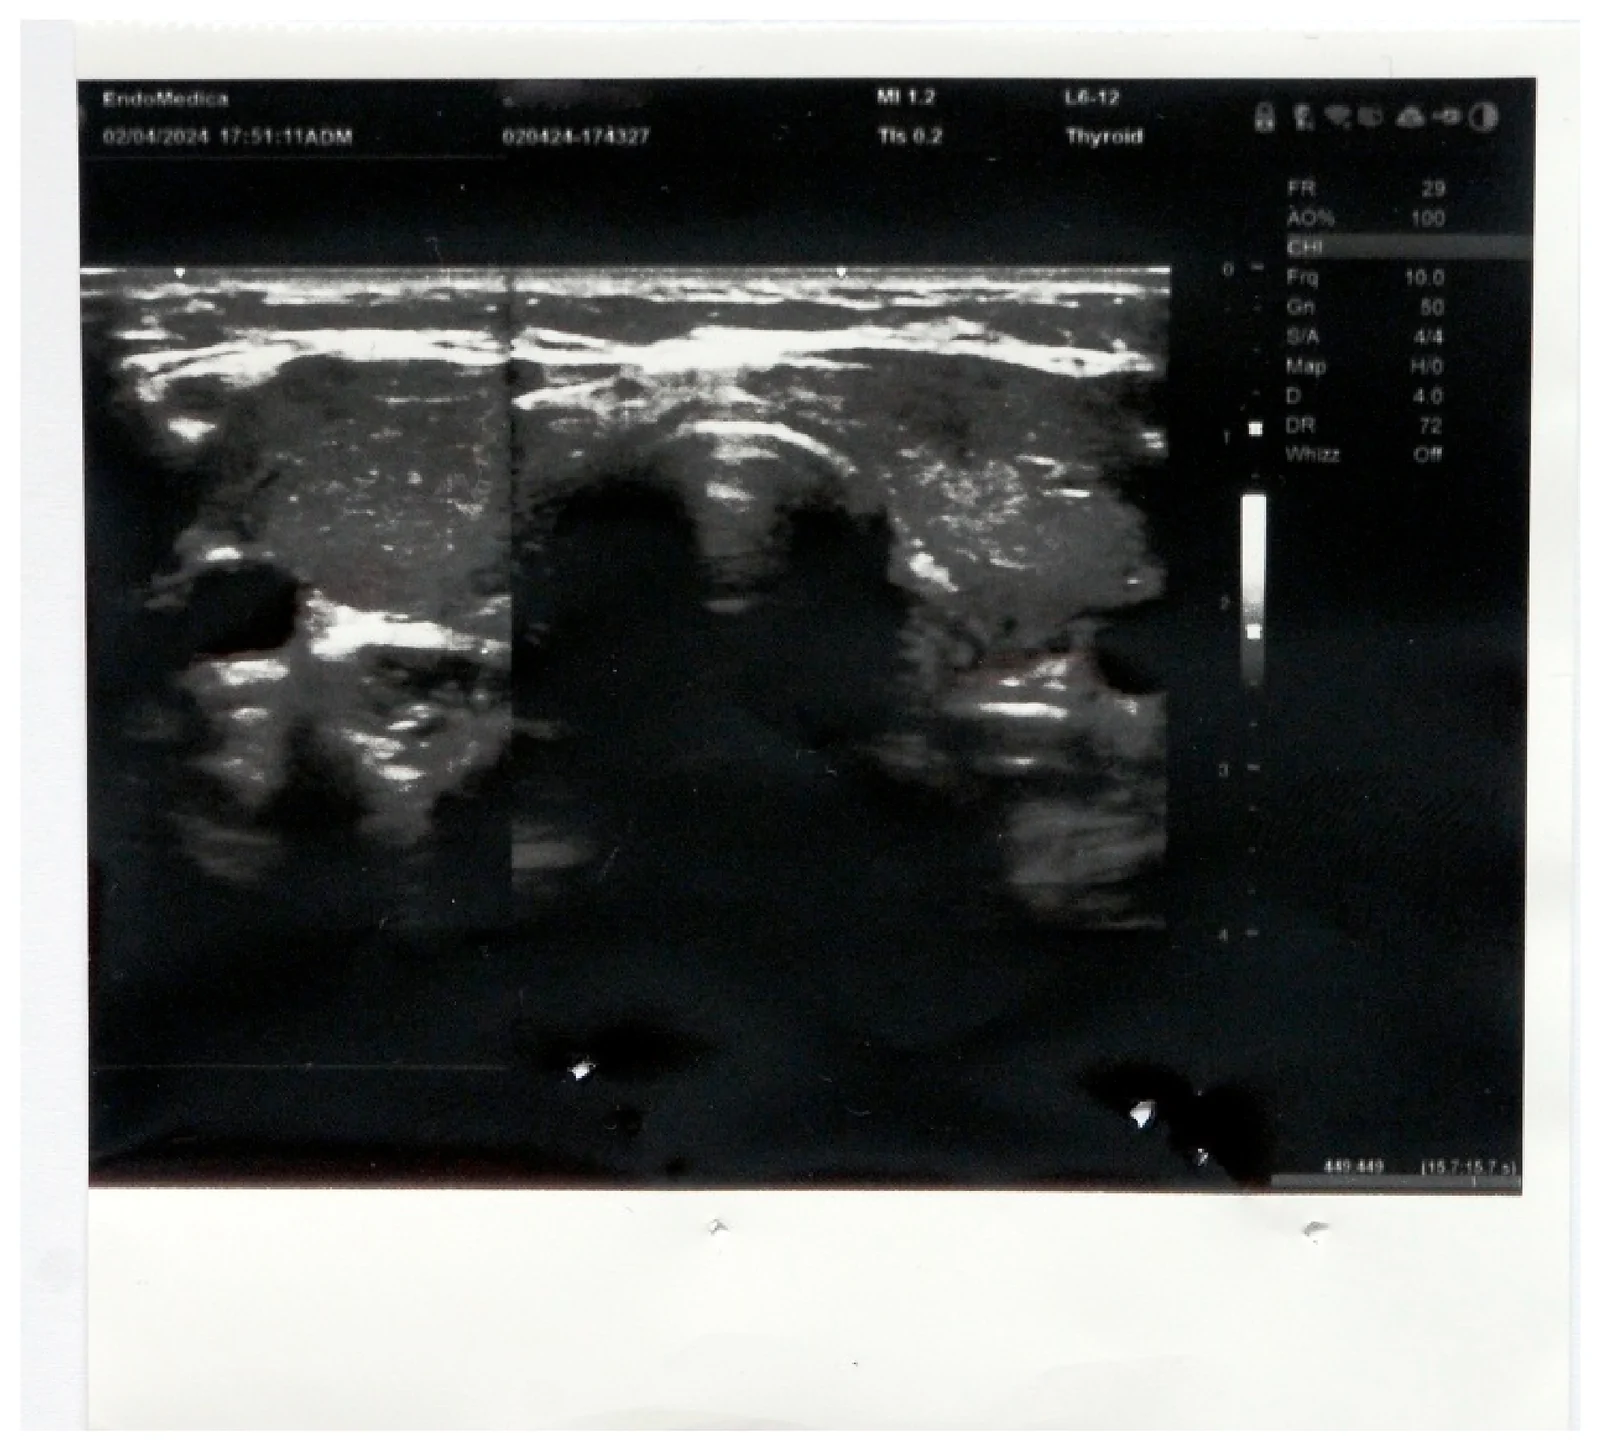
Neck ultrasonography (April 2024) showing enlarged thyroid lobes with heterogeneous hypoechogenic parenchyma, consistent with autoimmune thyroiditis. Isthmus: 1.2 × 0.37 × 2.6 cm.
